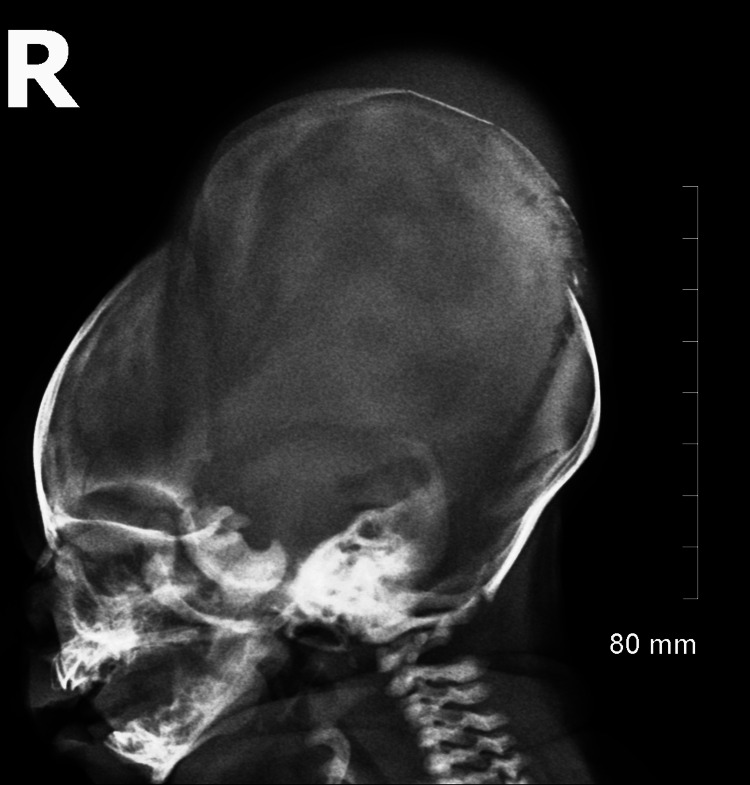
Lateral view x-ray image of the neonate within hours of delivery.
